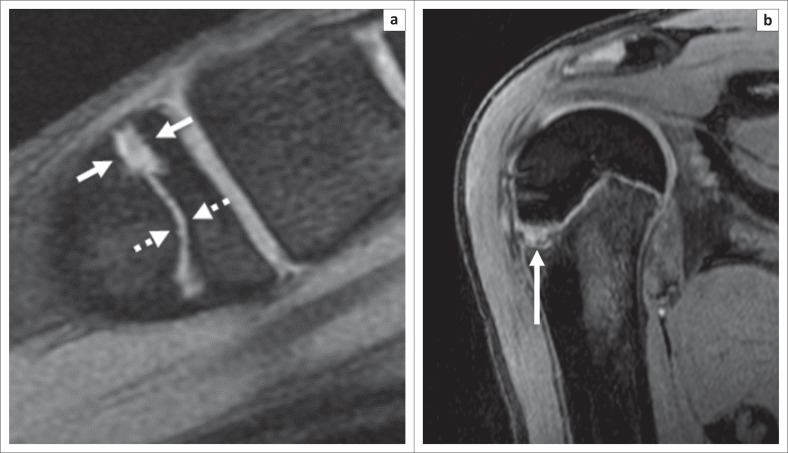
Chronic physeal stress on magnetic resonance imaging. (a) Sagittal gradient echo magnetic resonance image (959/14 ms, 20° flip angle) of the great toe in the same girl delineates abnormal widening of the dorsal physis (solid arrows) relative to the normal plantar physis (dotted arrows). The signal intensity is similar between the normal and the abnormal portions of the physis. (b) Sagittal volumetric gradient echo magnetic resonance image (13.9/5.2 ms, 30° flip angle) of the proximal right humerus of a 12-year-old male baseball player shows a focal region of abnormal widening (arrow) of the lateral aspect of the proximal humeral physis, with a configuration similar to the great toe metatarsal physis in the patient shown in Figure 2. This so-called ‘Little Leaguer’s shoulder’ is another example of physeal stress change attributed to a chronic traction injury.
